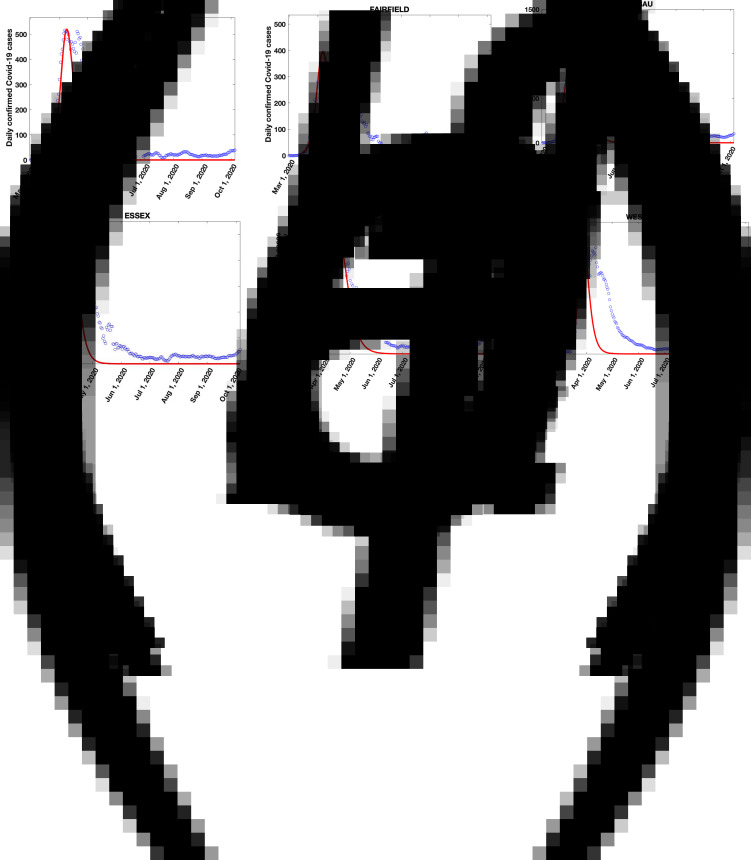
(a–f) LtoR top, LtoR bottom: Seven-day averaged daily Covid-19 cases (blue circles) in the two counties with the highest population densities in each of the tri-states: Hudson and Essex in NJ, Fairfield and New Haven in CT and Nassau and Westchester in NY. The solid red curves are fits to the data for the first peak from a SIR model (Methods and [30]).
